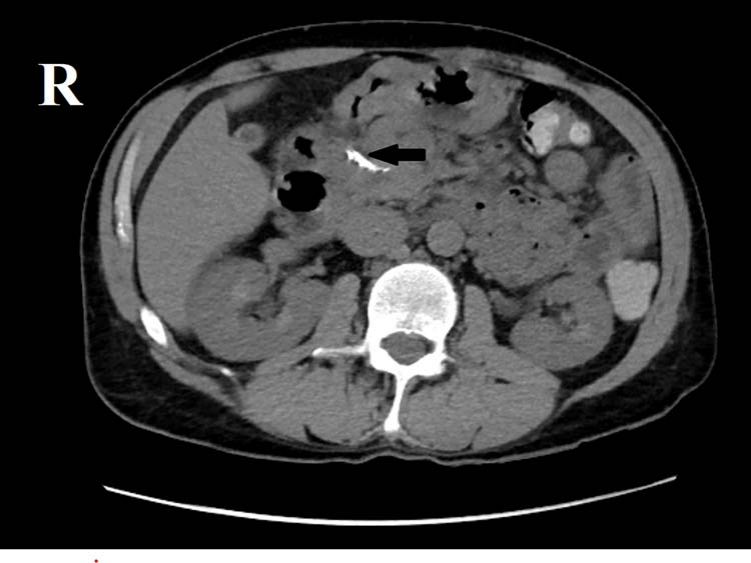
CT scan of pancreas (without contrast).
